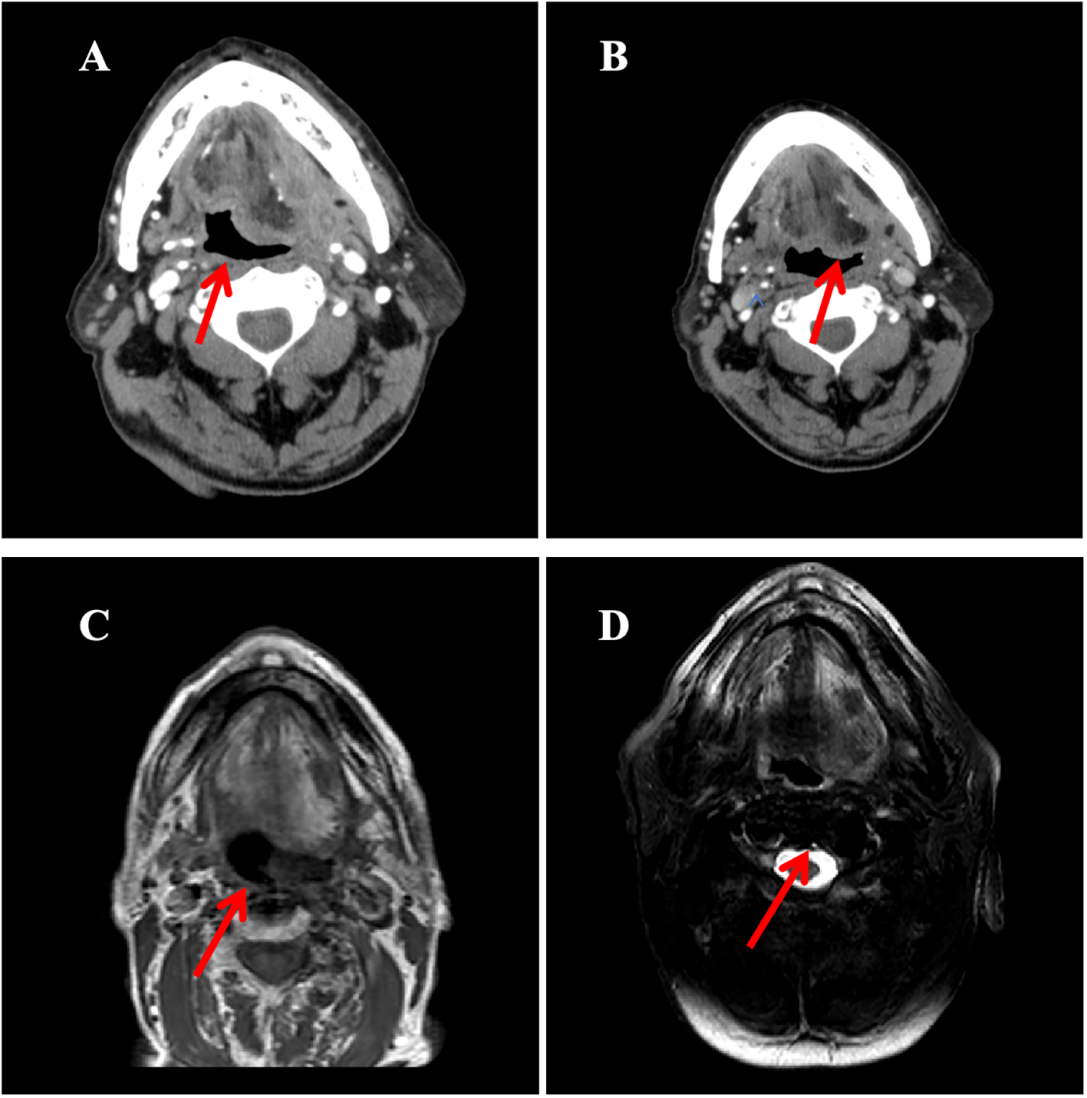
The imaging evolution of a left submandibular mass in a patient during the treatment period from November 2021 to October 2023. (A) Contrast-enhanced neck CT (October 2021): Demonstrates an enhancing mass in the left submandibular region (maximum diameter: 5.0 cm); (B) Contrast-enhanced neck CT (February 2022, After 4 cycles of treatment): Shows significant reduction in the size of the mass compared to A (maximum diameter: 3.2 cm); (C) Contrast-enhanced MRI of the submandibular gland (axial T1-weighted image; March 2023): Reveals a mass in the sublingual region (maximum diameter: 1.7 cm); (D) MRI of the submandibular gland (axial T1-weighted image; June 2023): The mass measures 1.8 cm in maximum diameter, indicating size stability compared to C.
